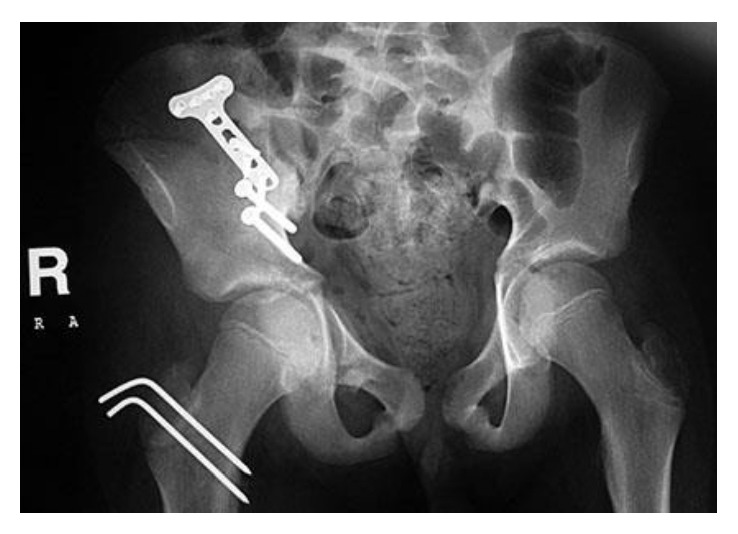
Postoperative AP pelvic X-ray showing adequate open reduction internal fixation.
